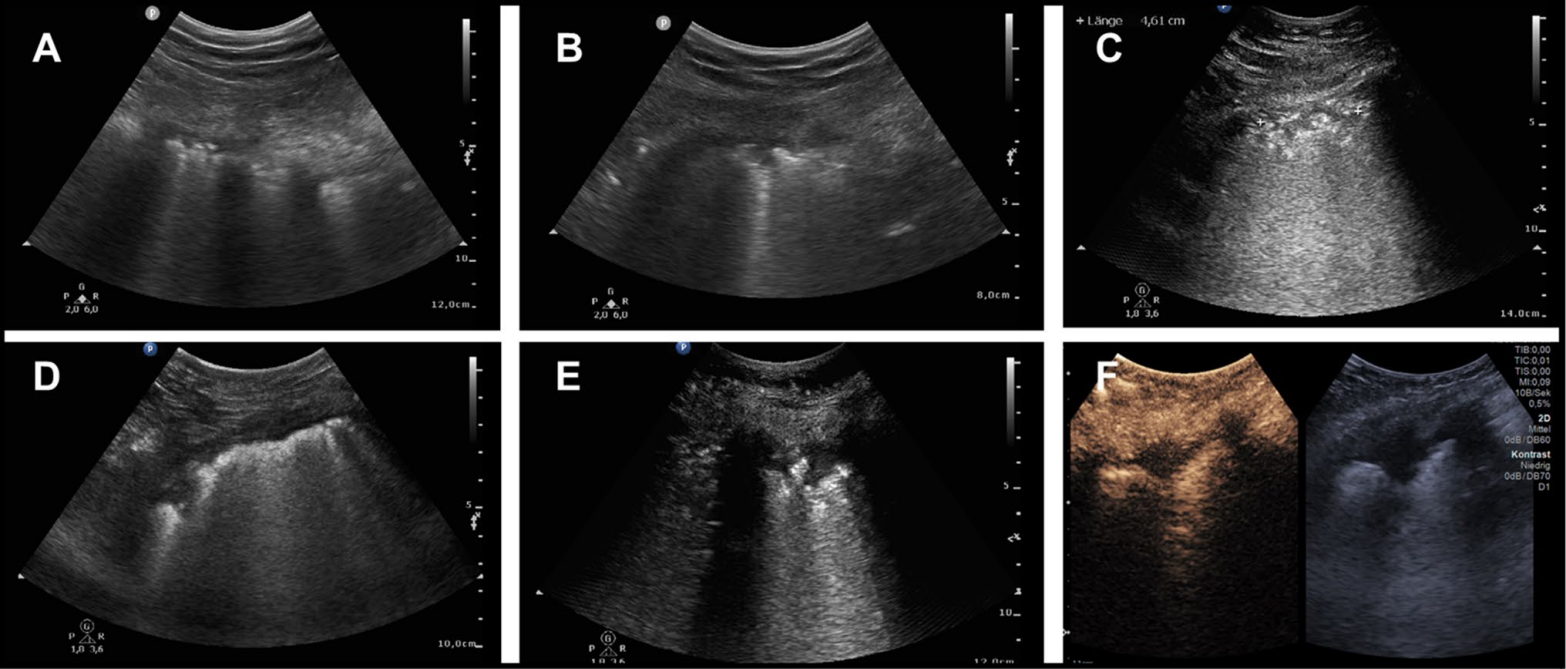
Sonographic image-examples of the pleural and subpleural changes in COVID-19 patients. a Typical thickening and irregularity of the pleura. b Small triangular subpleural consolidation < 1 cm. c Non-typical polygonal subpleural consolidation. d, e PE-typical triangular subpleural consolidation > 1 cm. f PE-typical triangular subpleural consolid.  > 1 cm, with additional documentation after contrast agent
